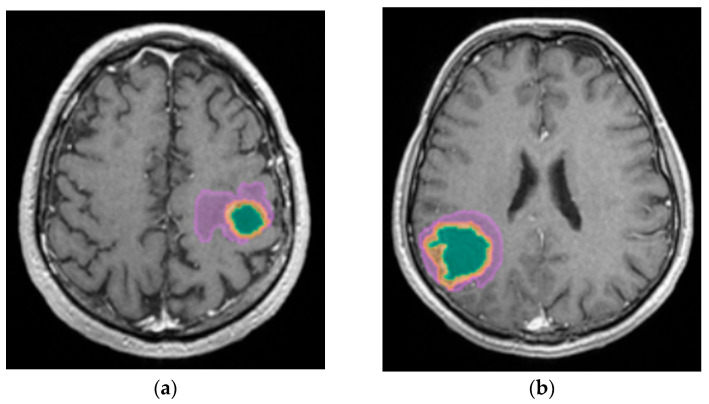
Mdbrain processing of MRI brain acquisition (DICOM). (a) T1W CE +FLAIR (Scale 1:24), axial plane, green area corresponding to the cystic portion of the lesion; (b) T1W CE + FLAIR (Scale 1:20), axial plane, green area corresponding to the necrotic center (approval was obtained from the Ethics Committee of the University of Medicine and Pharmacy “Grigore T. Popa” Iasi).
